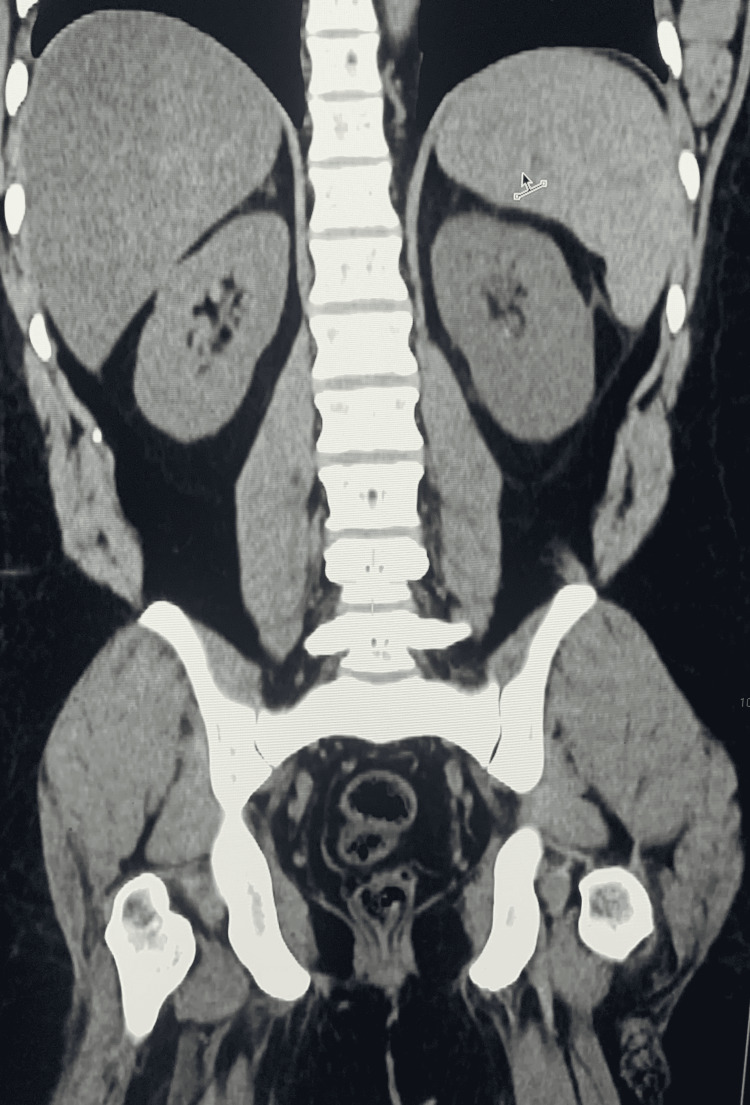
CT scan of abdomen (coronal view without contrast) shows: liver is enlarged with diffuse fatty infiltration; gallbladder is normal without any intraluminal calculus; adrenals and pancreas are unremarkable; spleen is enlarged and measures 26 cm with homogenous texture.
